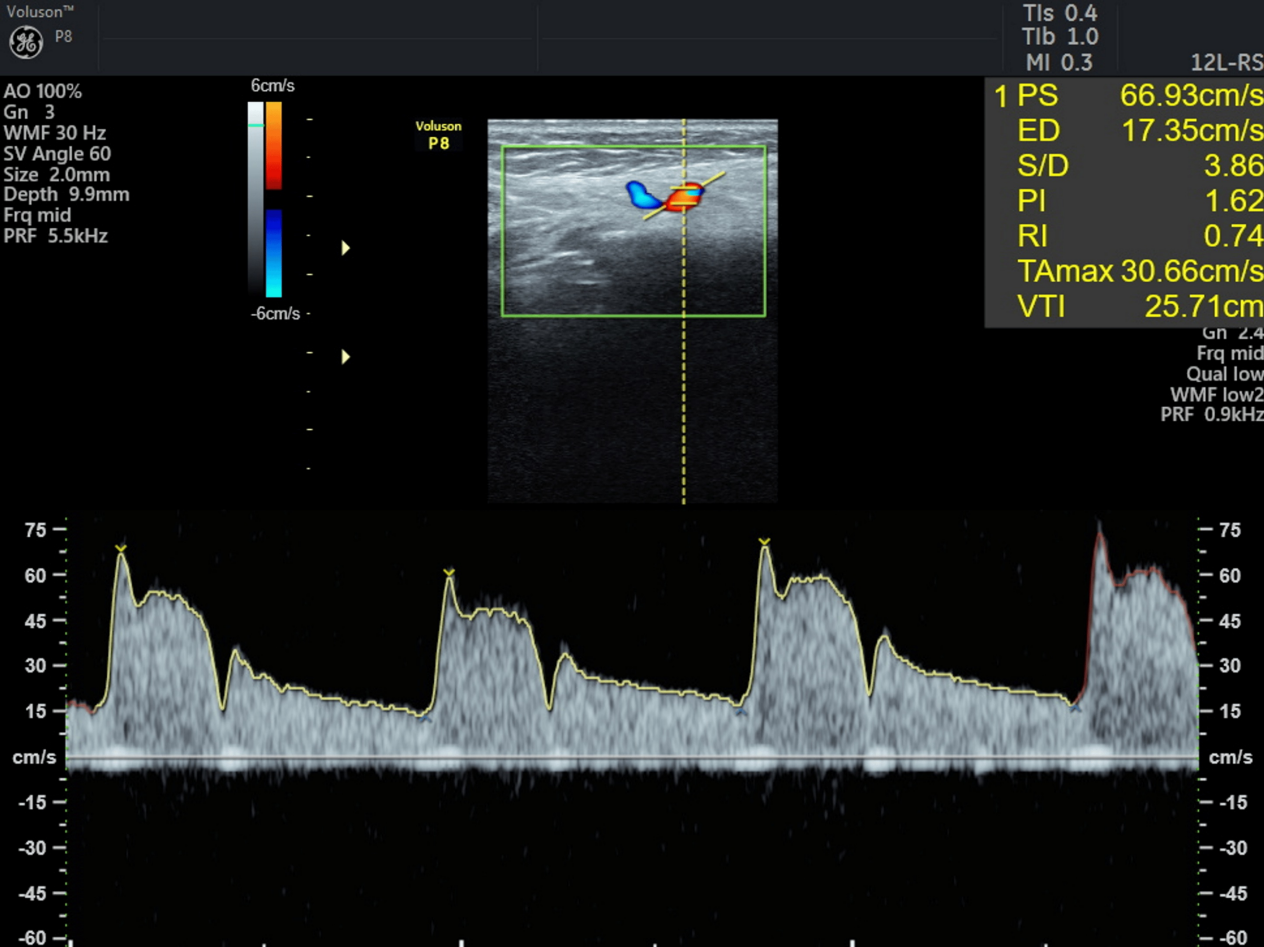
Ultrasound Doppler flowmetry of CAF + CTG on the third day. CAF: coronally advanced flap, CTG: connective tissue graft, PS: peak systolic velocity, ED: end-diastolic velocity, PI: pulsatility index, RI: resistive index, VTI: velocity time integral.
